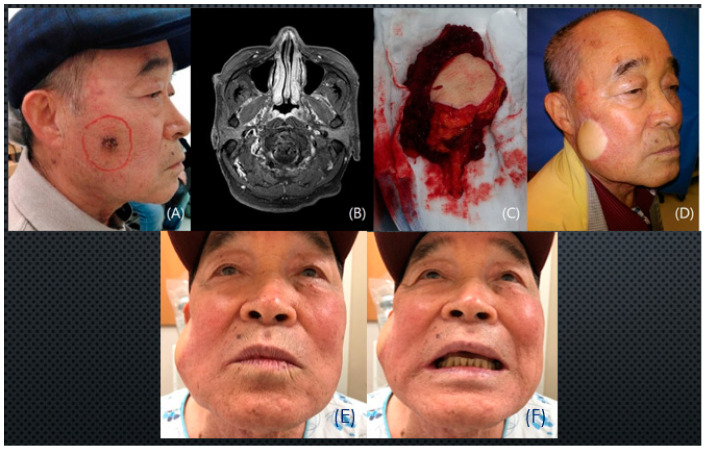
A case of a cheek squamous cell carcinoma patient. (A) Pre-operative photo. (B) Pre-operative MRI finding of facial nerve involvement; (C) 5 × 5 cm anterolateral thigh chimeric musculocutaneous flap for facial reanimation; (D) 11-month postoperative photo; (E,F) 11-month postoperative photo; the frontal view shows dynamic functional restoration.
